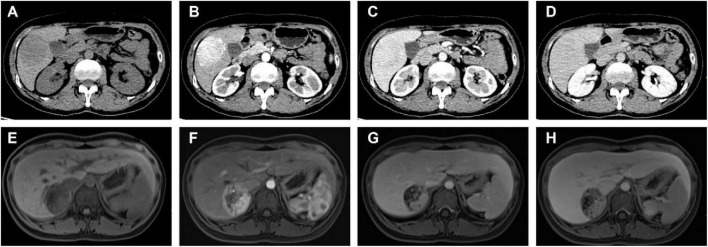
Representative CT and MRI findings from some patients. (A–D) CT images of a 51-year-old female patient: (A) Non-contrast scan shows a roundish, slightly hypodense mass in the inferior segment of the right hepatic lobe, slightly protruding beyond the liver contour, measuring approximately 6.5 × 4.5 cm. (B) Arterial phase contrast-enhanced scan reveals heterogeneous marked enhancement, with a CT value of approximately 114 HU. (C) Portal venous phase and (D) delayed phase images demonstrate washout of contrast, with enhancement slightly lower than that of the normal liver parenchyma. (E–H) MRI images in a 17-year-old female patient. (E) A roundish lesion exhibiting long T1 and long T2 signals is observed in the right posterior liver lobe, measuring 48 × 42 × 46 mm (transverse × craniocaudal dimensions). (F) Arterial phase post-contrast imaging shows obvious heterogeneous enhancement. (G) Portal venous phase and (H) delayed phase images reveal enhancement of the solid components similar to that of the adjacent liver parenchyma.
